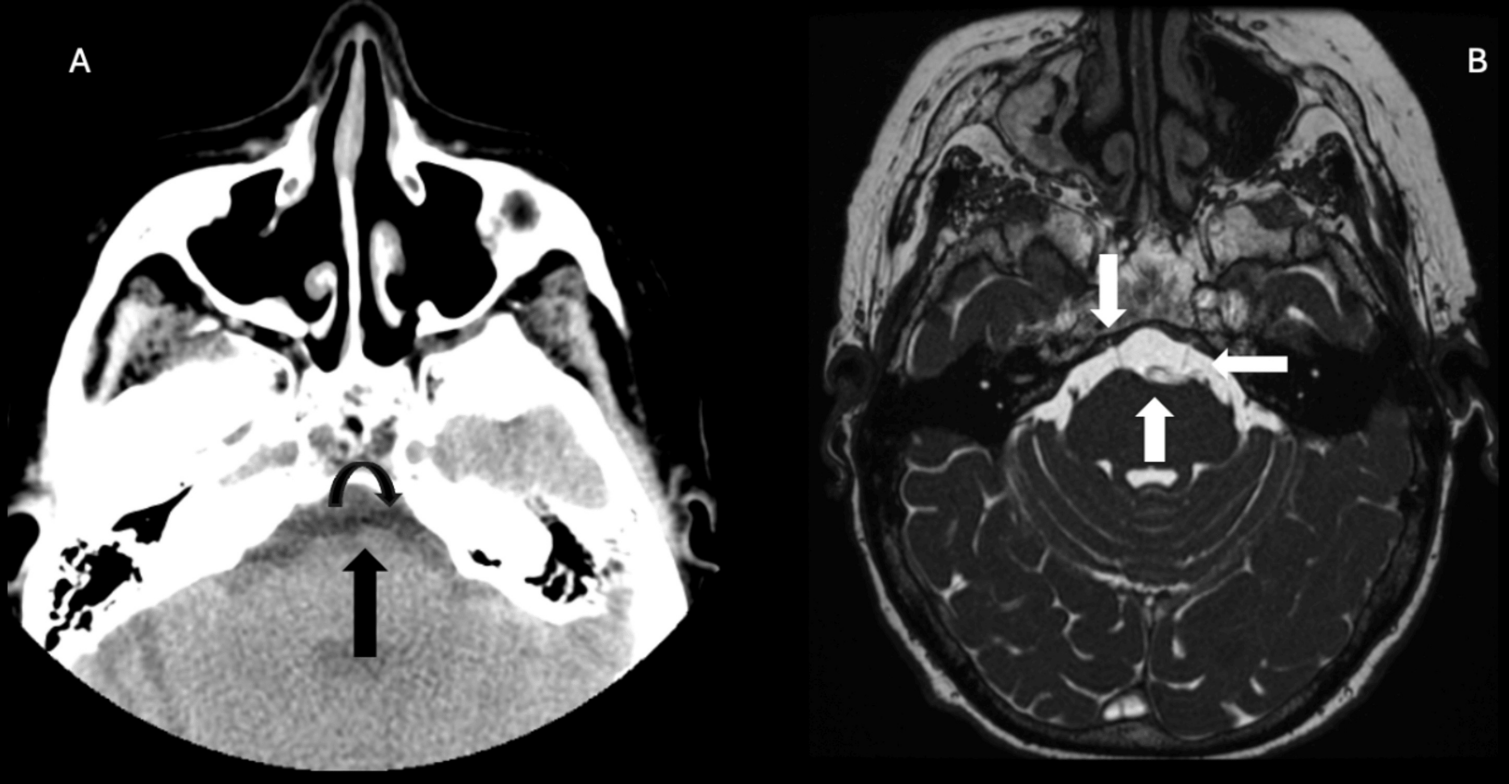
A) Axial CT scan shows the basilar artery intending the left sixth nerve at the exit zone (the vertical arrow shows the basilar artery and the curved arrow shows the left sixth nerve). B) The FIESTA sequences show the basilar artery with indentation of the brain stem at the exit zone (the up arrow shows the basilar artery, the down arrow shows the normal right sixth nerve, and the left arrow shows the compressed left sixth nerve).
